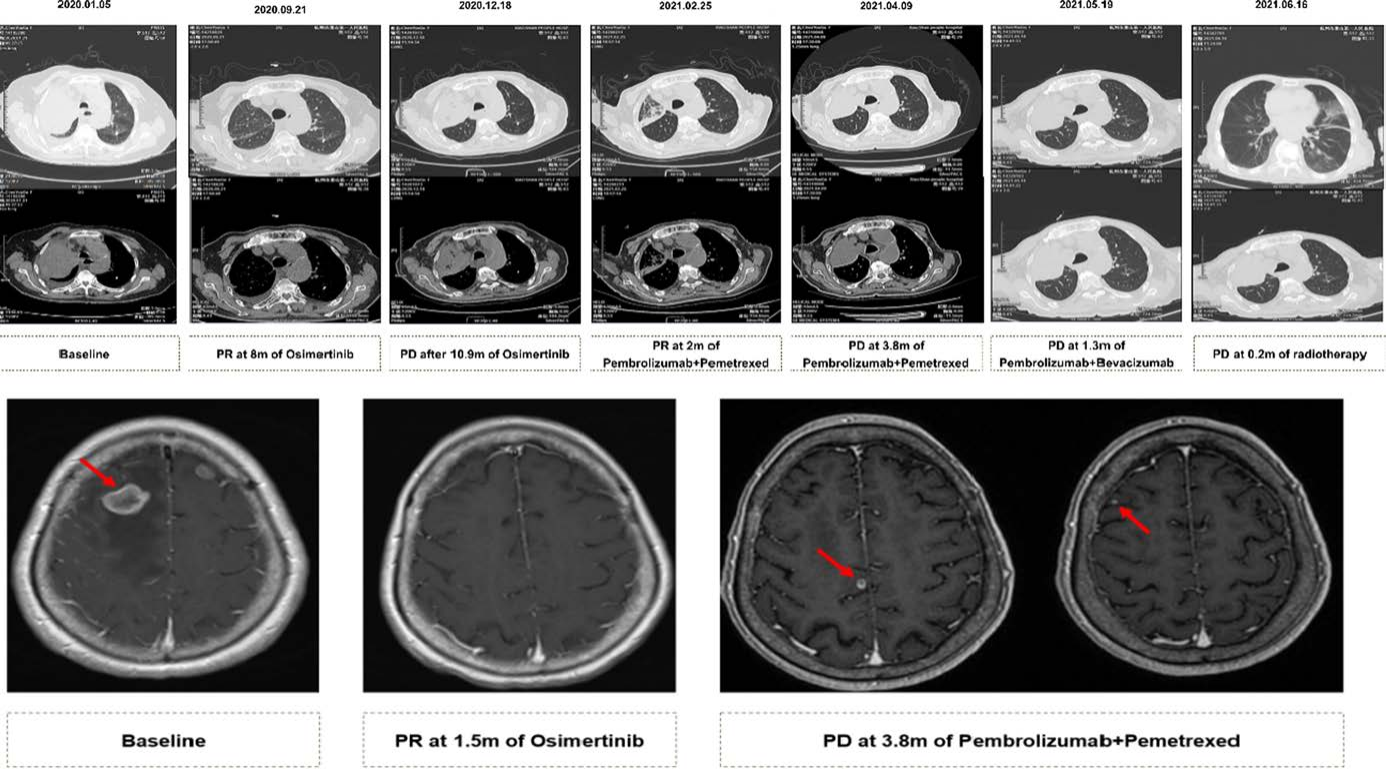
Representative CT images and MRI images at indicated time points, shown along with corresponding treatment regimen and efficacy evaluation.
